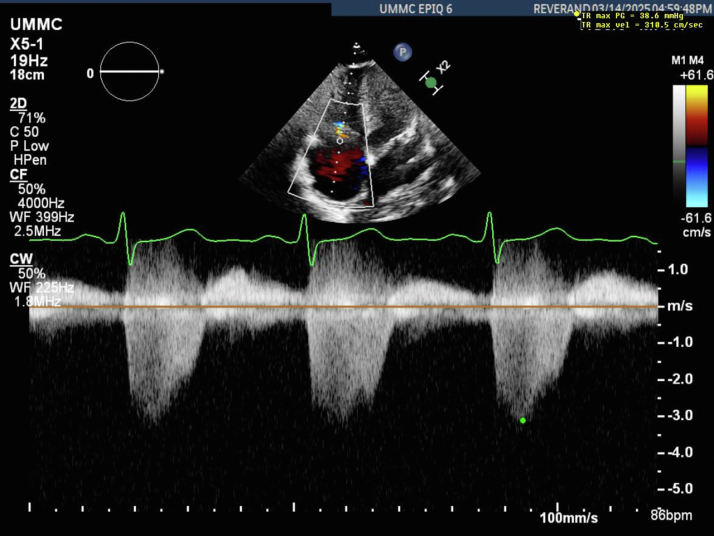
Transthoracic Echocardiography at 22 Weeks Gestation Patient presented at 22 weeks gestation with dyspnea on exertion. Tricuspid valve regurgitation with a TR max velocity of 320.0 cm/s, correlating with a right ventricular systolic pressure of 49 mm Hg. TR max velocity = tricuspid regurgitation maximum velocity.
